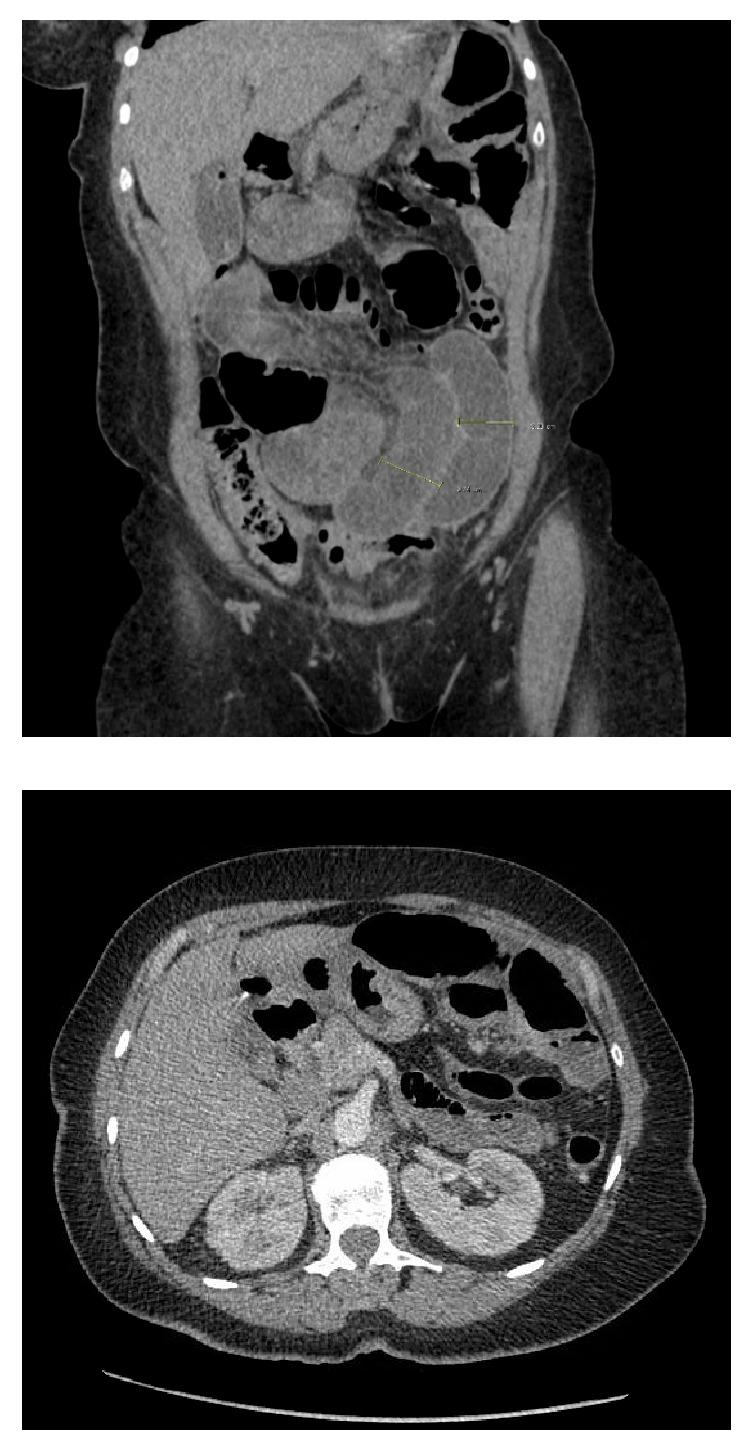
Computed tomography (CT) scan of the abdomen demonstrated early complete bowel obstruction at the level of midjejunum.
